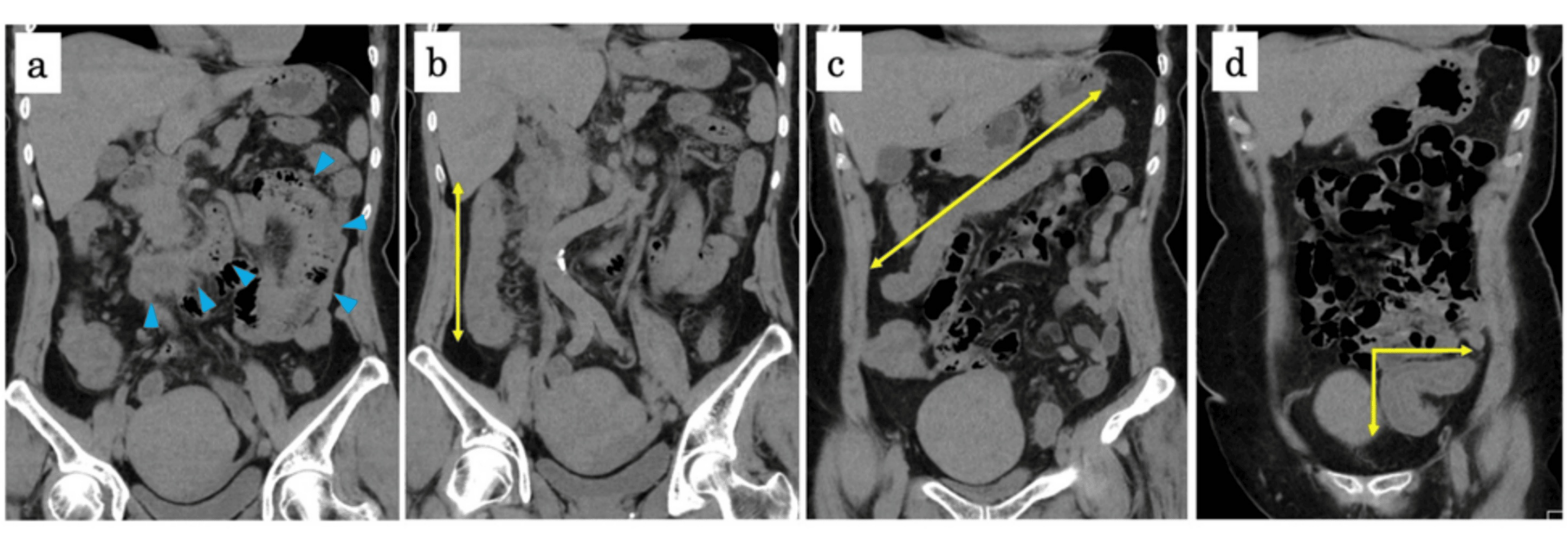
CT on admission Abdominal CT showing thickened wall of the transverse part of duodenum and proximal jejunum (a, blue arrowheads), ascending colon (b, yellow arrow), transverse colon (c, yellow arrow), and sigmoid colon (d, yellow arrow).
